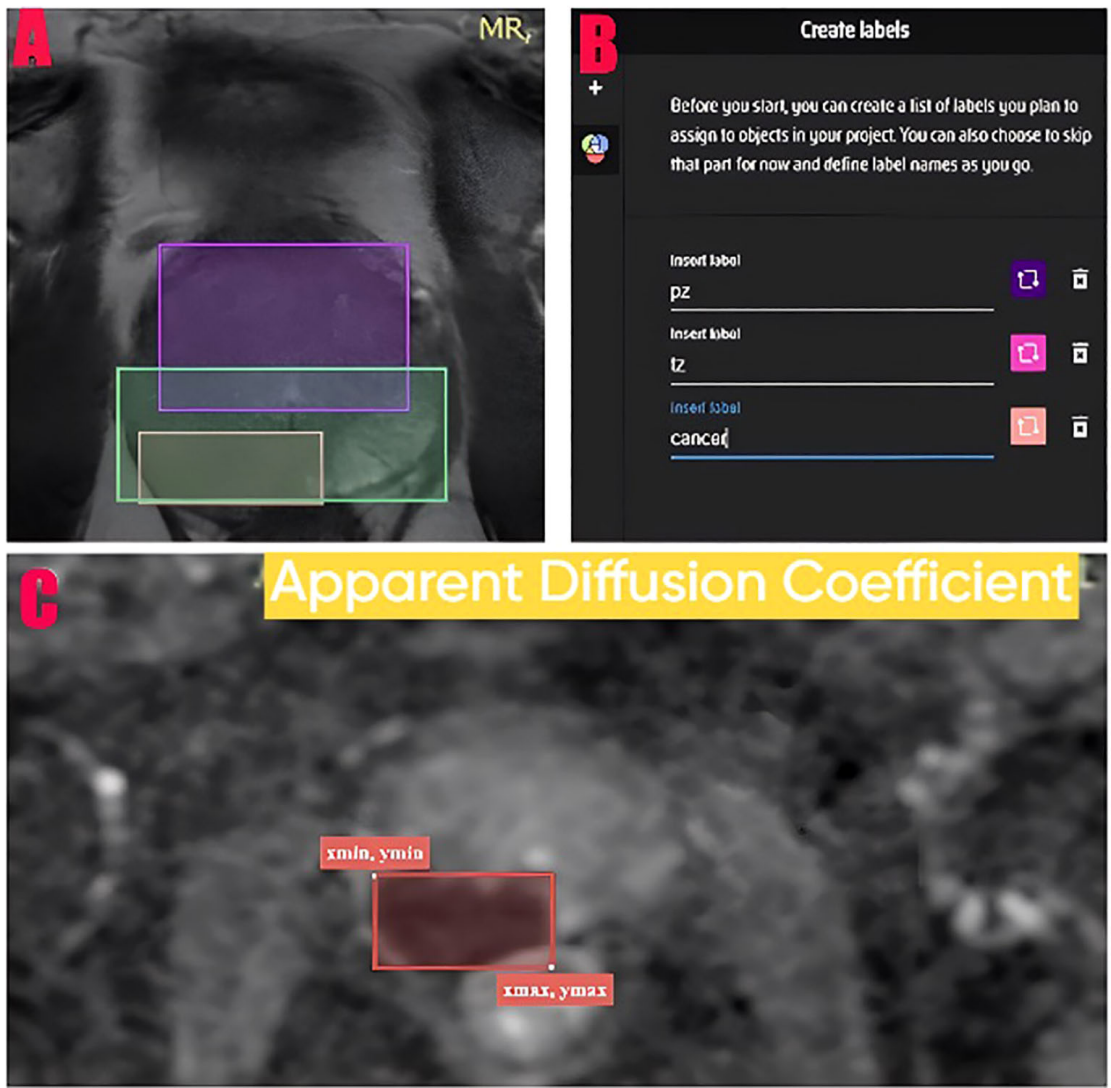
Data labeling methodology and coordinate system. (A) The axial T2 MR image displays multiple labels for peripheral zone (pz - purple), transitional zone (tz - pink) and cancer (cancer - orange). (B) The MakeSense platform interface and label creation panel. (C) The ADC image shows a coordinate system which displays the xmin, ymin (top left corner) and xmax, ymax (bottom right corner) coordinates of the label. The image coordinate system begins at the top left corner (0,0) and extends to the right and downwards.
